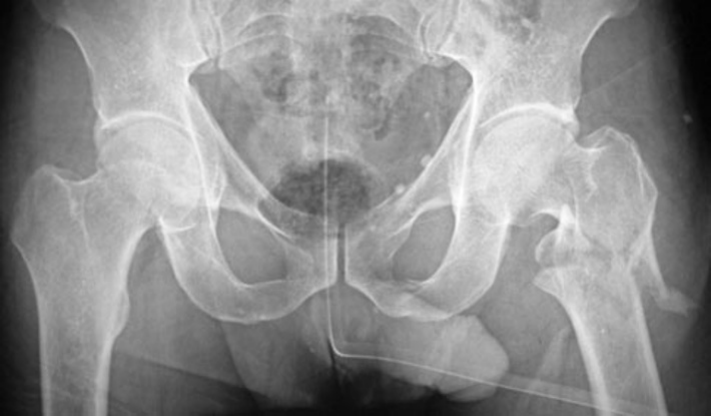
Preoperative radiograph of the fractured left femur
